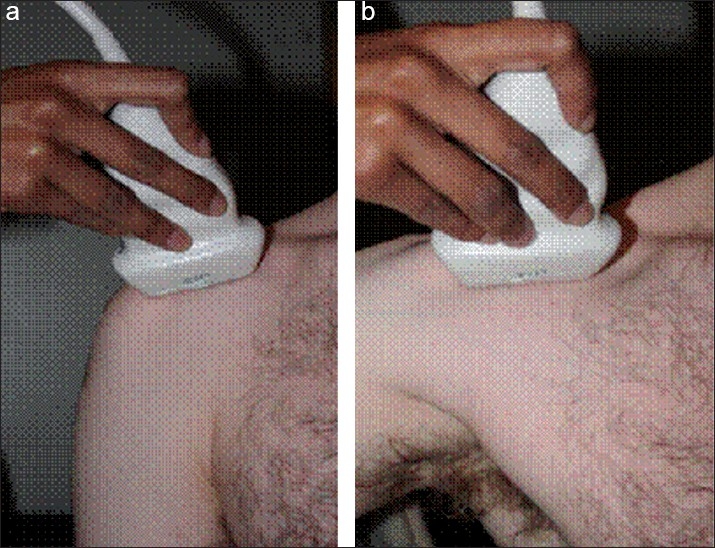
Clinical photographs demonstrating the ultrasound probe position, with the arm at the side (a), and on active abduction (b)
